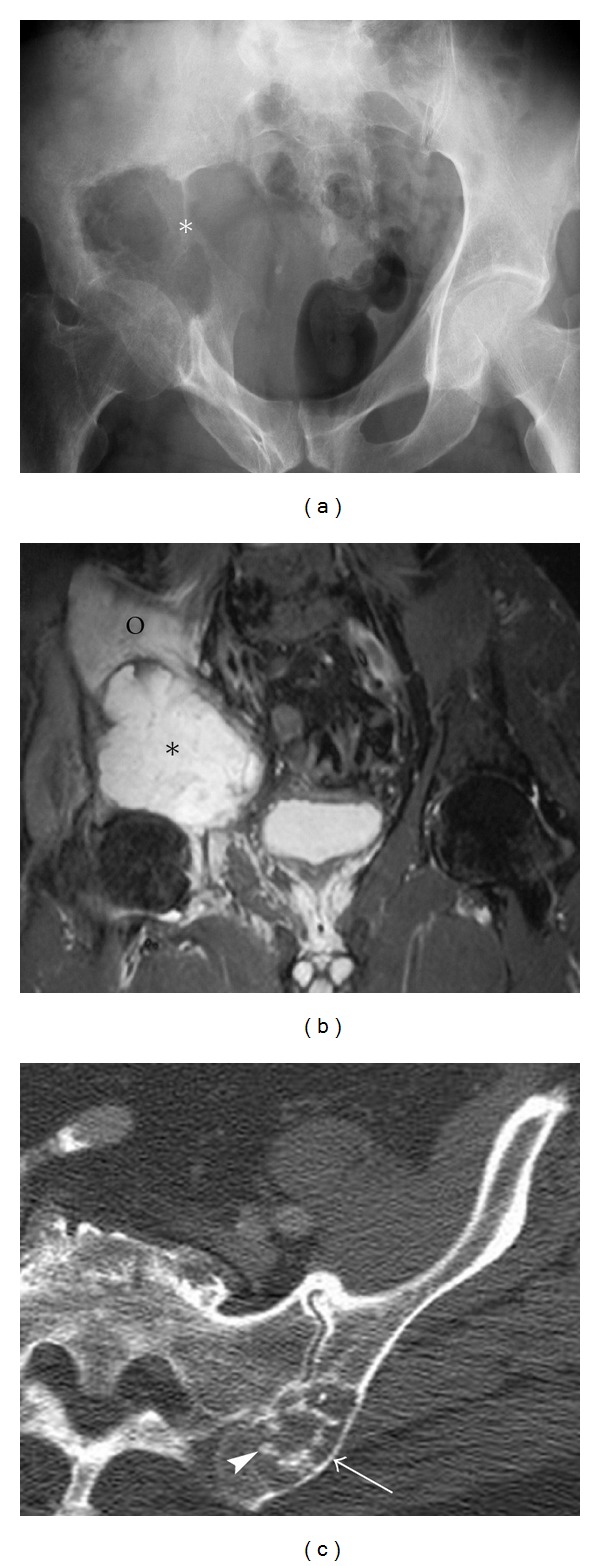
Chondrosarcoma. A 62-year-old male presents with right hip pain, diagnosed with chondrosarcoma. Pelvic radiograph (a) outlines a lytic destructive abnormality centered on the superior acetabular region (asterisk). An associated soft tissue mass is seen extending into the pelvis. The relative lack of calcification of the chondroid matrix would be in keeping with a high-grade chondrosarcoma. Coronal STIR (b) demonstrates the destructive mass (asterisk) as well as associated marrow edema within the right iliac bone (circle) and a small right hip joint effusion. Axial CT scan of another patient demonstrates more typical appearances of chondrosarcoma with endosteal scalloping (arrow) and chondroid matrix (arrowhead) (c).
